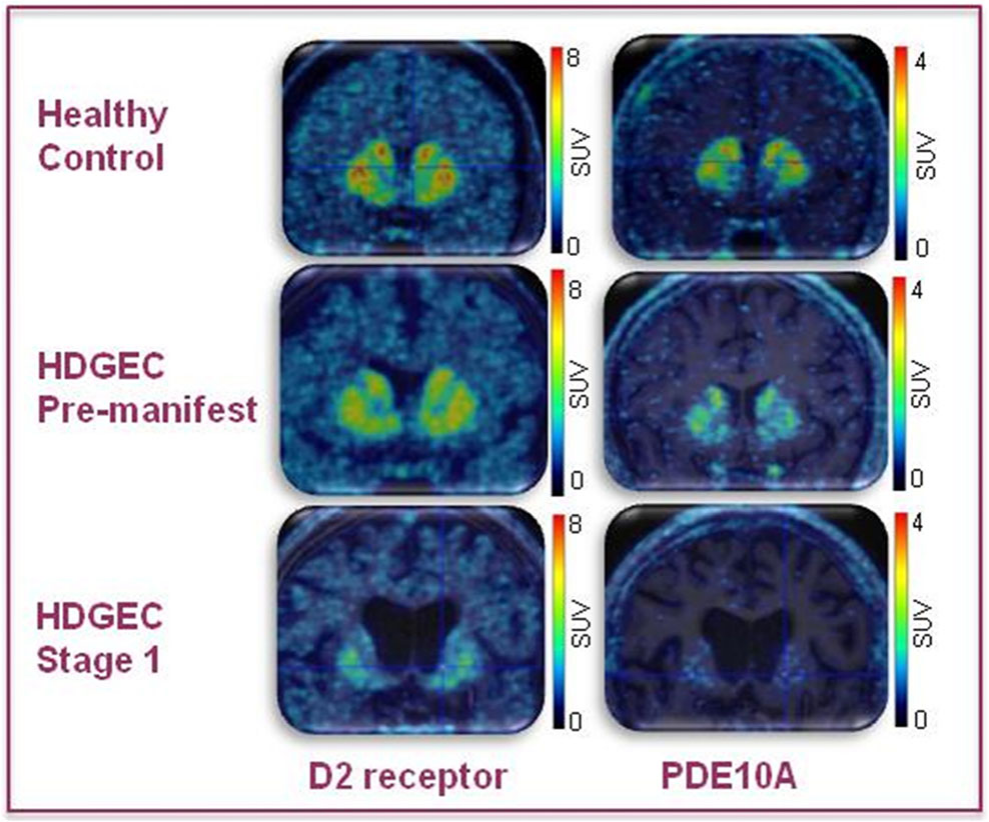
Representative standardized uptake values (SUV) PET images (D2 receptors and PDE10A enzyme) depicting coronal brain section at the level of basal ganglia for HD mutation carriers at different stages of the disease overlaid on top of individual MRI image
